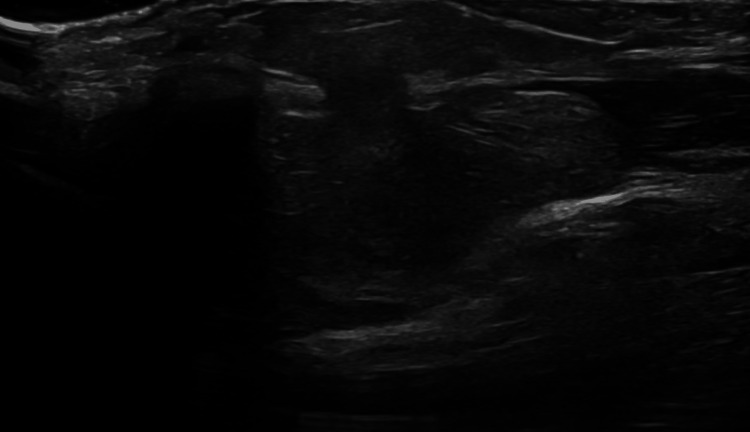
Ultrasound image of the left wrist showing a subcutaneous lobulated soft tissue mass on the dorsal ulnar side, measuring 1.5 cm x 2 cm x 2.5 cm. The lesion is well-encapsulated with homogeneous echotexture. A superficial component is radially located in relation to the intact extensor carpi ulnaris tendon, while a deep component extends toward the underlying distal radioulnar joint space.
